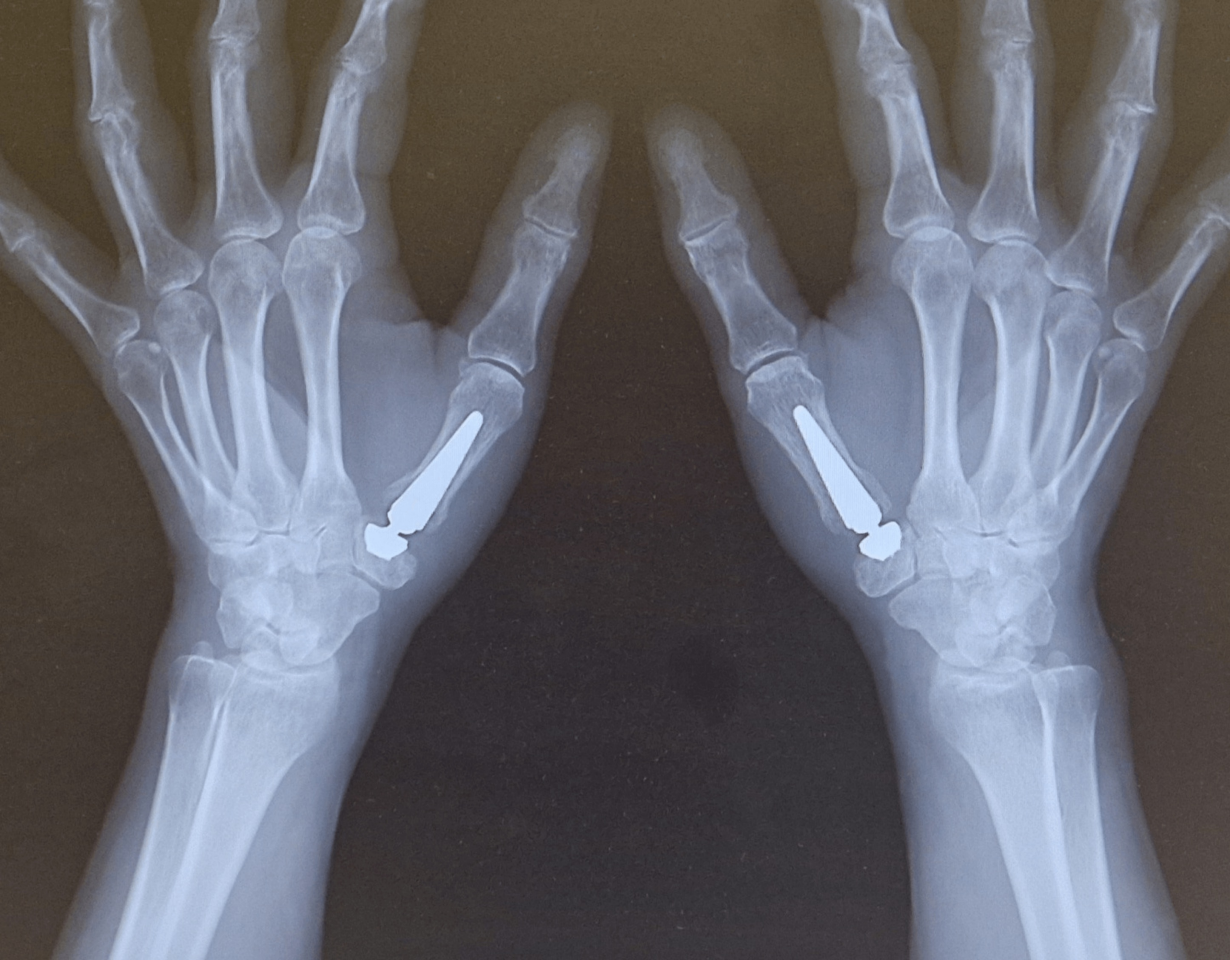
Postoperative X-ray in the Kapandji projection
